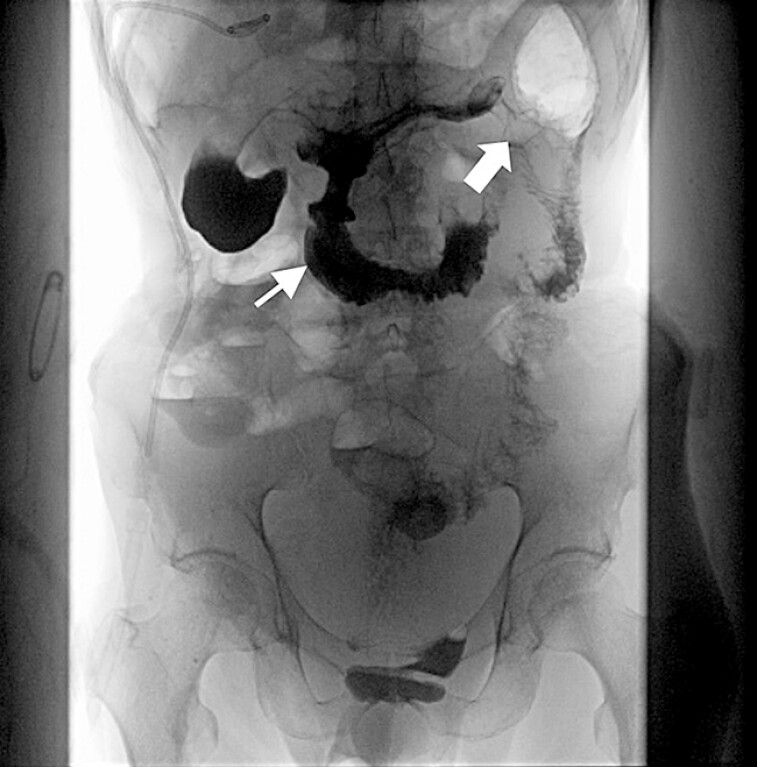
Postoperative radiography demonstrated stent patency after the secondary EUS-GE (thin arrow: contrast agent passed the initial stent but limited to the afferent limb; bold arrow: contrast agent passed the second stent and reached the distal jejunum).
